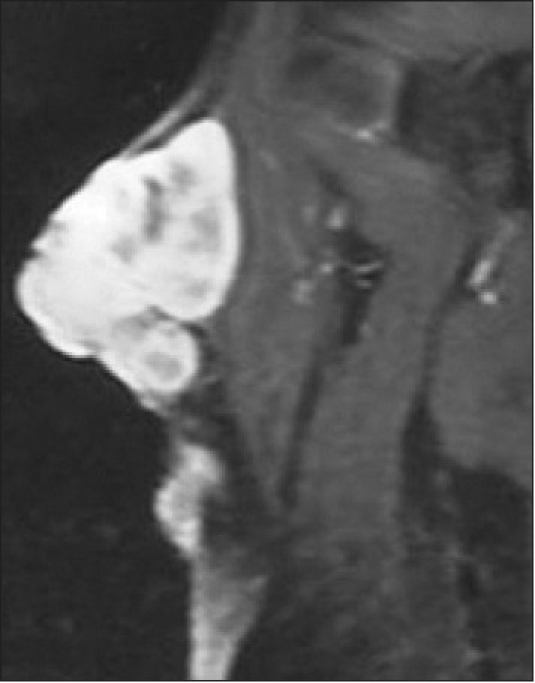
The T_2_-weighted, fat-saturated coronal MR image showed the solid mass with multilobulated, relatively homogenous high-signal-intensity pattern, with focal invasions in the deep muscle planes and cutaneus and subcutaneus tissues
